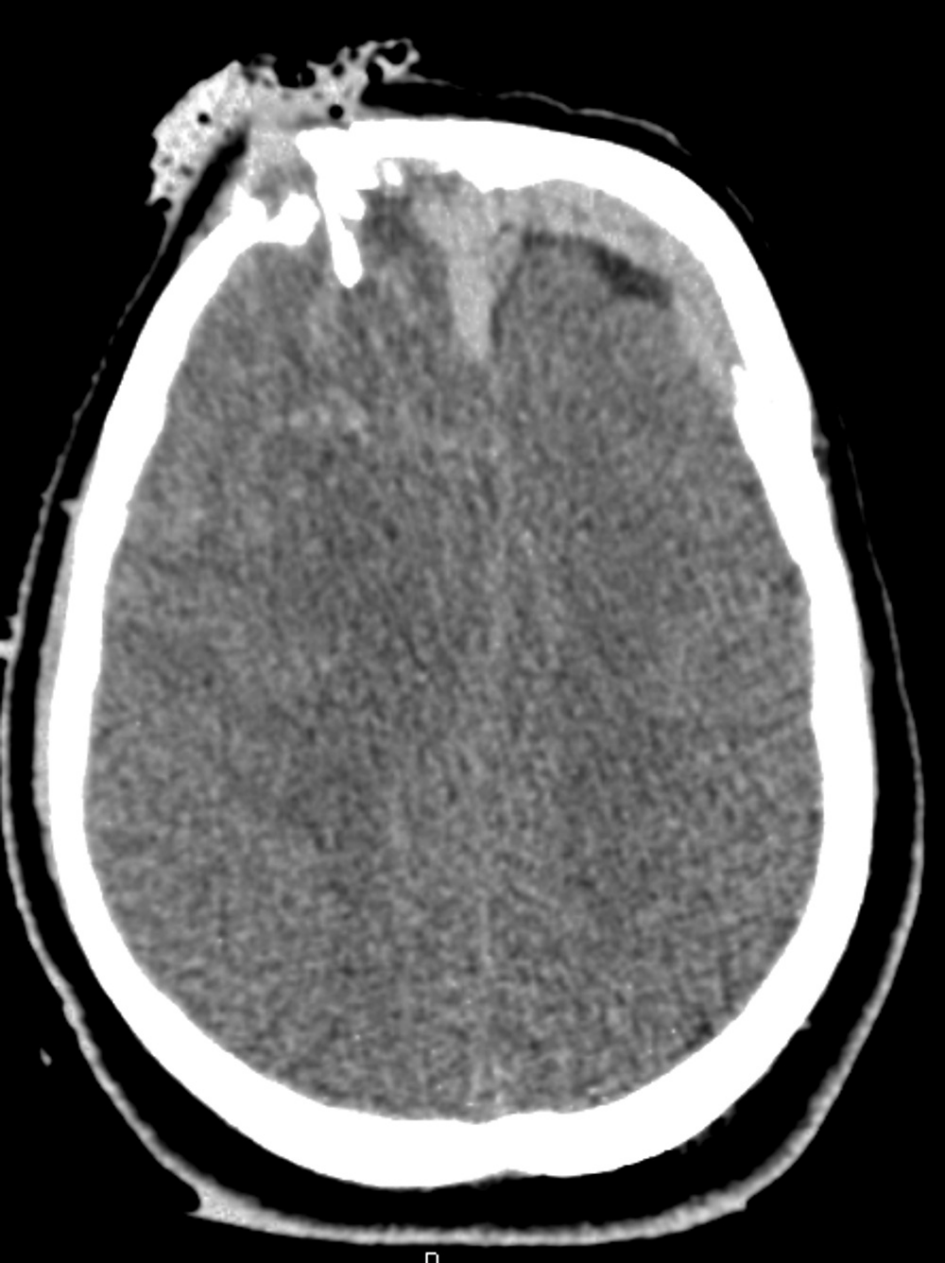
Preoperative CT head showing a depressed skull fracture and associated subdural hematoma
